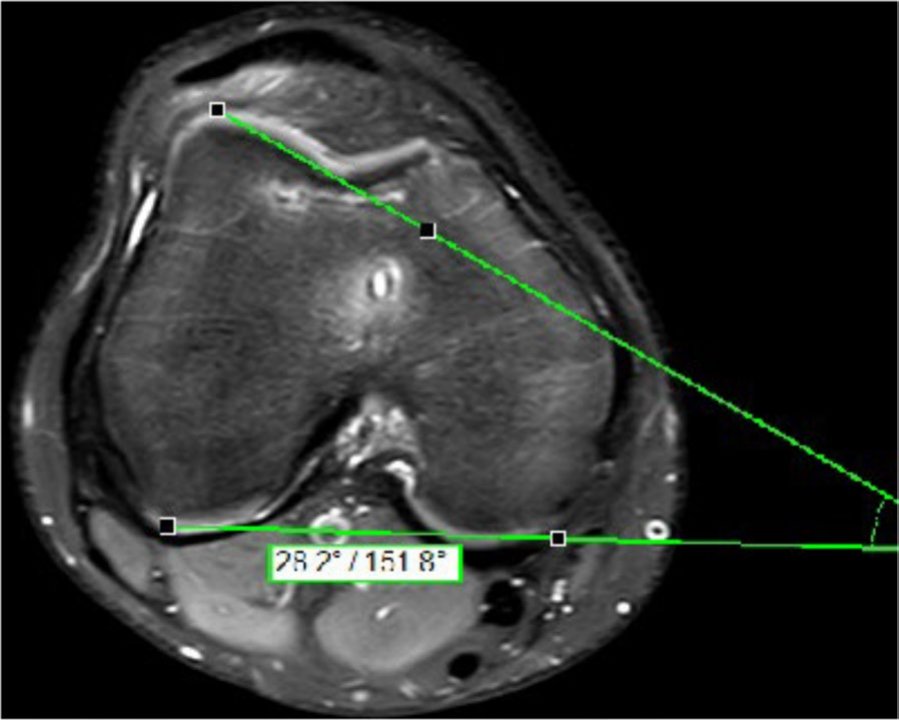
Measurement of the lateral trochlea inclination (LTI): The LTI is measured in the MRI slices 3 cm above the femorotibial joint space by measuring the angle between a line tangential to the subchondral bone of the posterior aspect of the femoral condyles and a line tangential to the subchondral bone of the lateral trochlear facet
